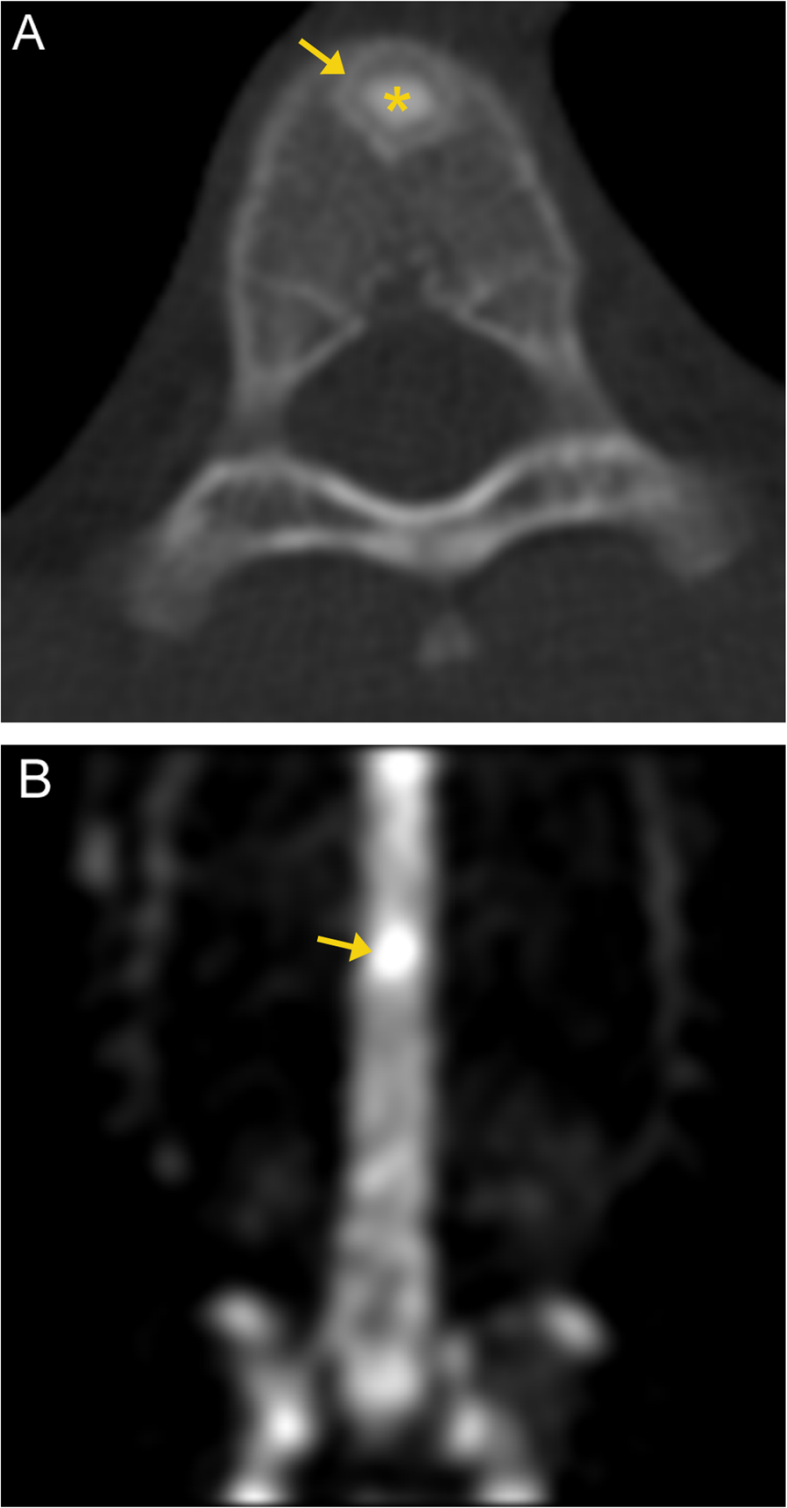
Osteoid osteoma. Axial CT image (a) demonstrates a 1.2-cm sclerotic nidus (asterisk) at T10. The lesion is sharply demarcated with surrounding reactive sclerosis (arrow). Nuclear medicine bone scan (b) demonstrates focal intense uptake at T10 corresponding within the lesion (arrow)
